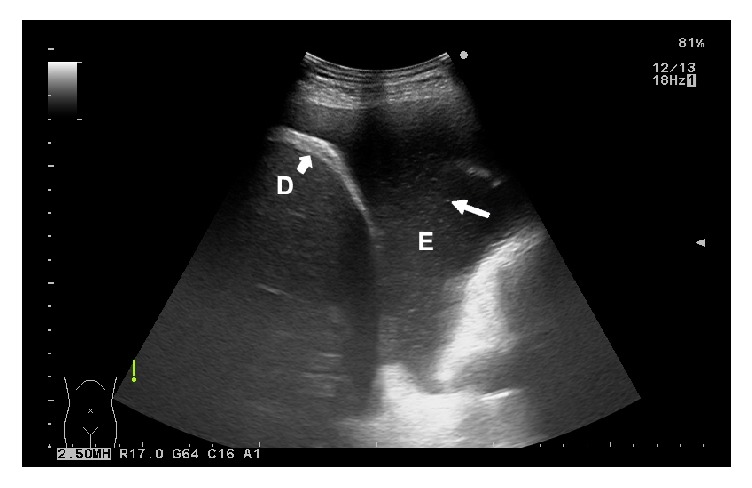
Typical ultrasonographic image of complex nonseptated malignant pleural effusion. Heterogeneously hyperechoic spots inside effusion (right white arrow) without fibrinous septations can be seen. D = diaphragm (left bold white arrow); E = pleural effusion.
